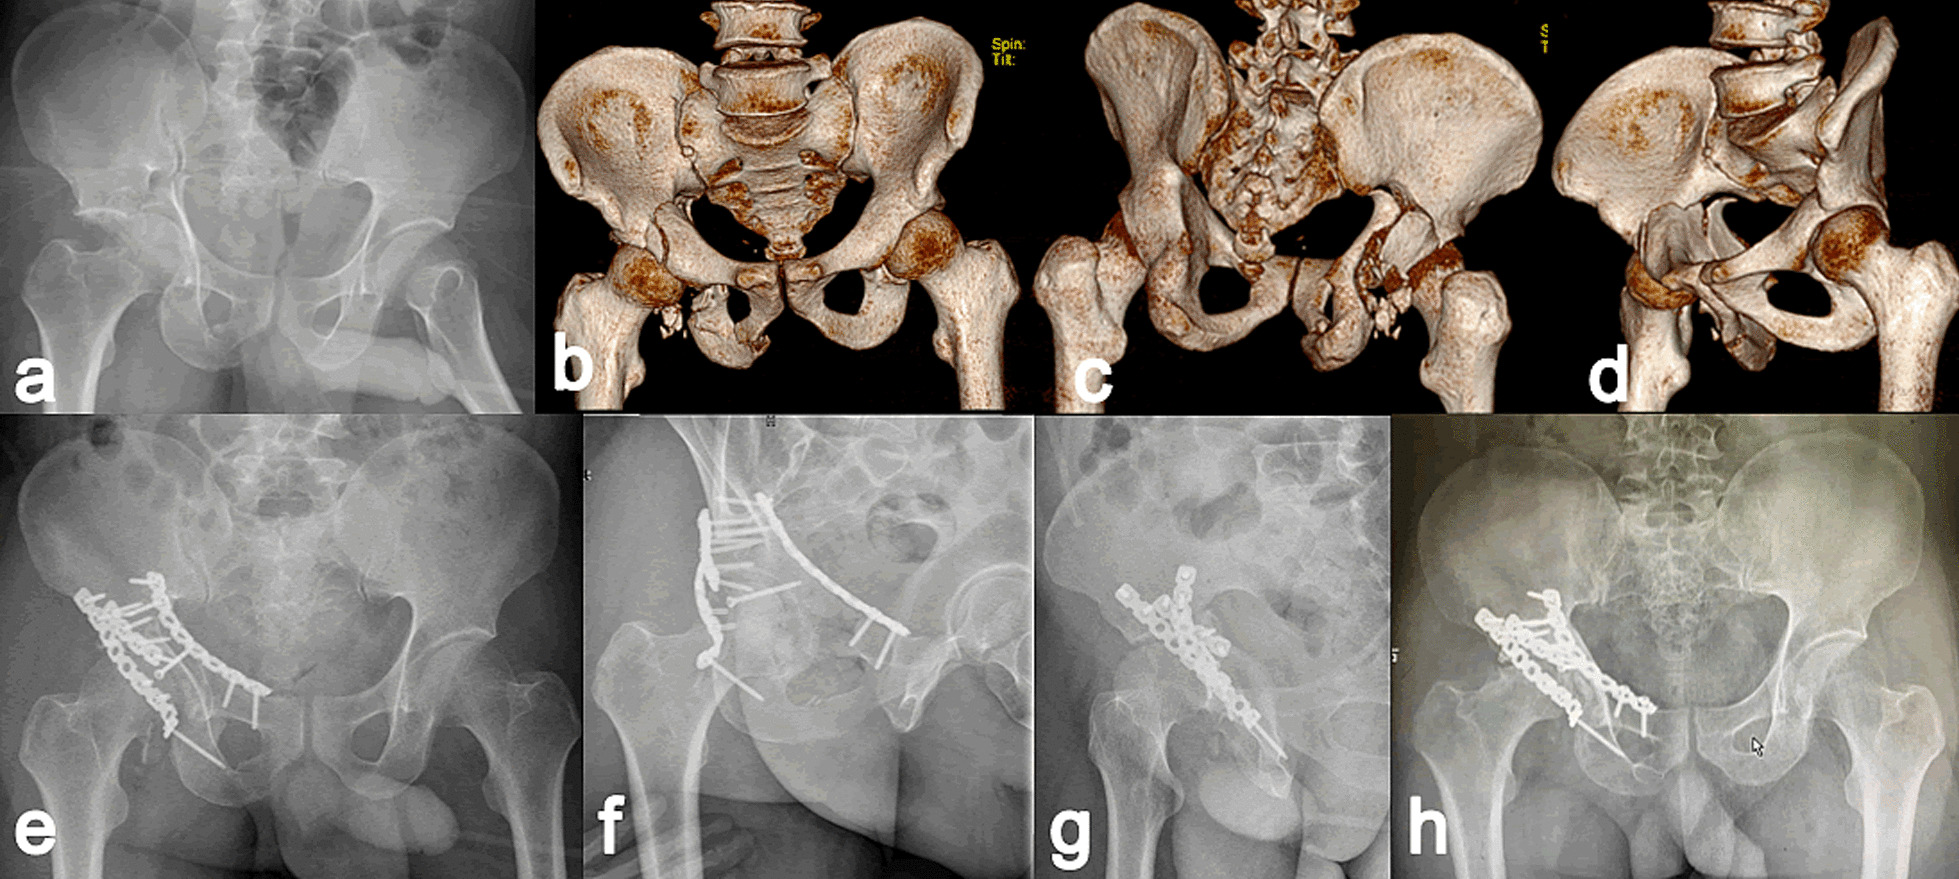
A 53-year-old male who sustained T-shaped acetabular fracture with the posterior wall treated with KL + Stoppa approaches 18 days after the traffic accident. a Preoperative anteroposterior radiograph; b–d Preoperative three-dimensional reconstructions; e–g Immediate postoperative radiographs showing imperfect reduction; h At 1 year of follow-up, the patient presented poor outcome with right hip pain and claudication due to avascular necrosis of the femoral head, which verified by postoperative radiograph
